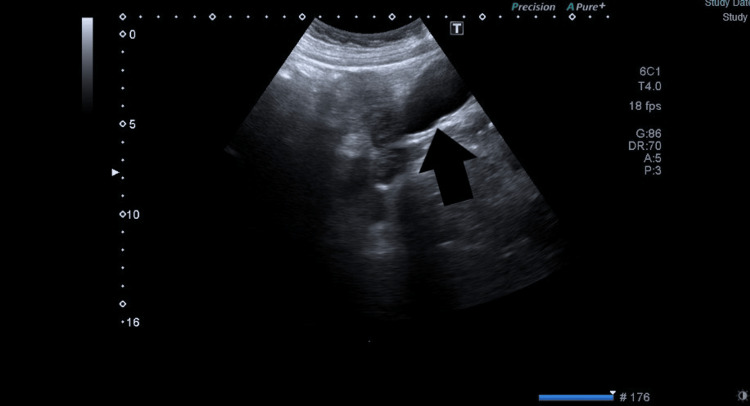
Ultrasound showing the gallbladder.
